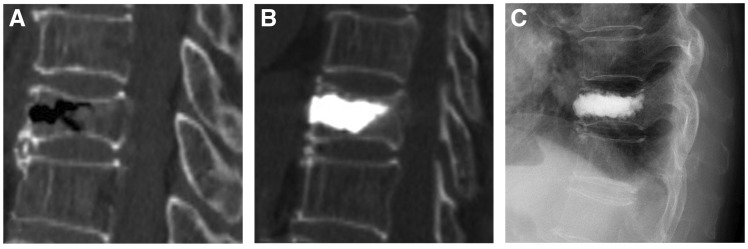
An 81-year-old male patient underwent PVP for T12 OVCFs. (A) Preoperative CT showed the compression fracture of the T12 vertebral body. (B, C) Lateral projection re-examination by CT and X-ray performed 3 days and 1 year postoperatively
